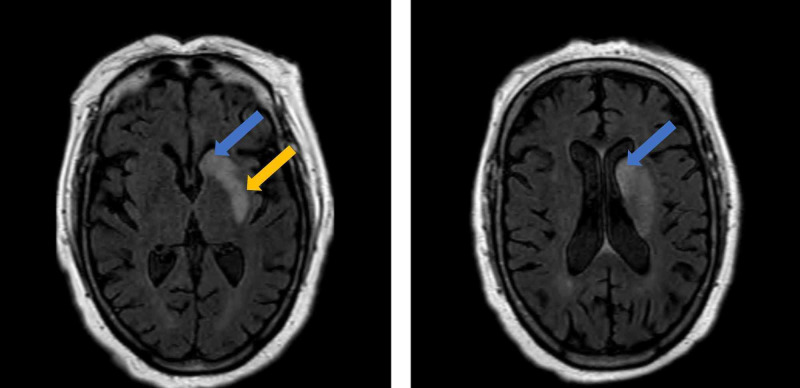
MRI showing an acute/sub-acute infarct involving the left caudate nucleus (blue arrow) and putamen (yellow arrow).
